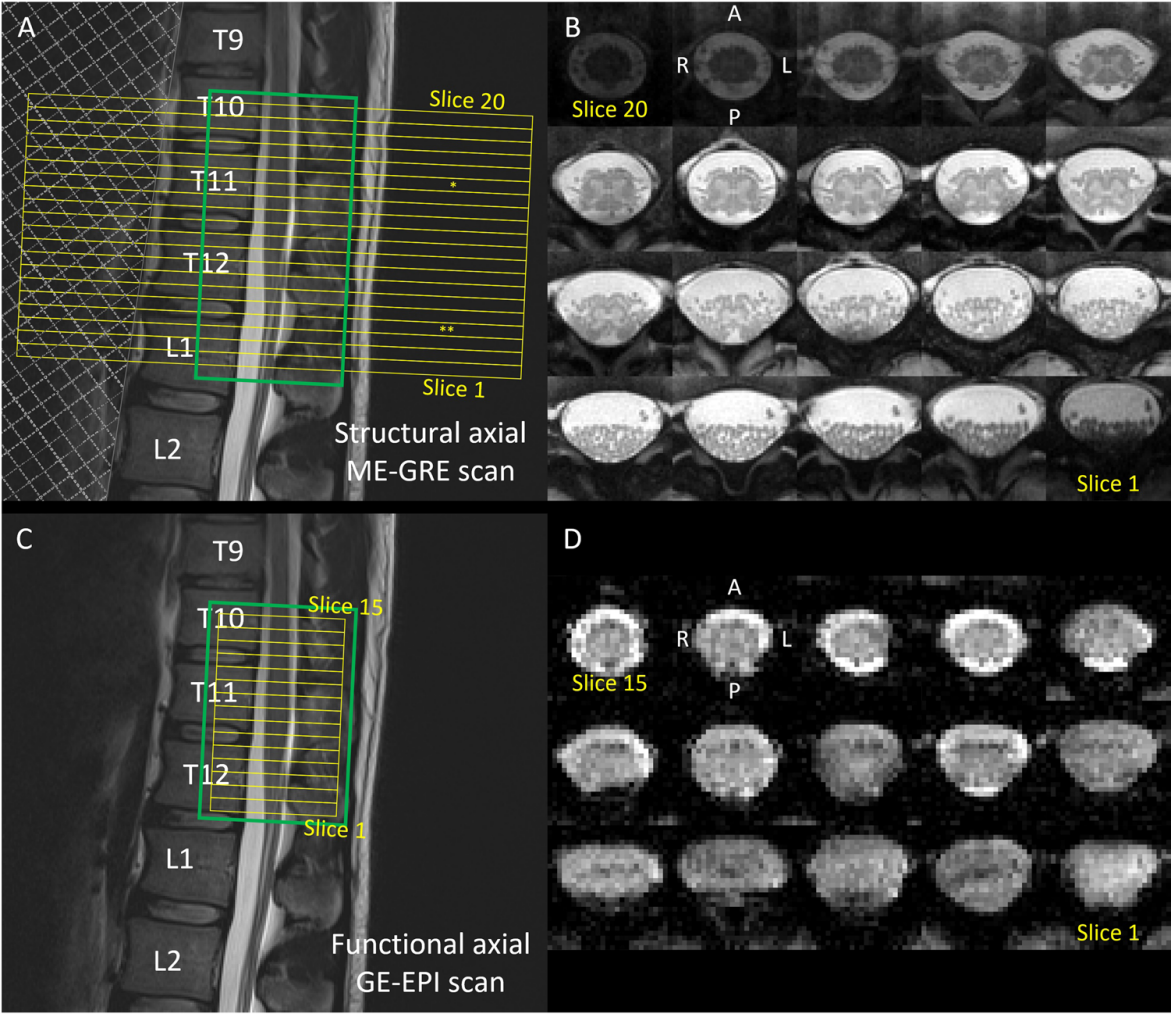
Slice positioning and example slices. Slice prescription was based on a sagittal T2-weighted turbo spin echo scan of the lower spine, for the (A) structural 3D spoiled multiecho gradient-echo (ME-GRE, Siemens FLASH) sequence and the (C) functional reduced field-of-view single-shot gradient-echo echo planar imaging (GE-EPI) sequences. The corresponding axial slices are shown for the (B) ME-GRE and (D) GE-EPI sequences (here, the iFOV35 version, seeTable 1), displayed in rostral (top left) to caudal (bottom right) direction. The slice stacks (20 slices for ME-GRE, 15 slices for GE-EPI) and shimming volumes are indicated by yellow and green boxes, respectively. The field of view (FOV) for the GE-EPI scan was set such that its sixth most rostral slice (slice #10) aligned with the maximum width of the spinal cord in the lumbosacral enlargement as observed in the sagittal T2-weighted image. The FOV for the ME-GRE scan was positioned to align the top edge with that of the GE-EPI scan (i.e., slice #20 in ME-GRE corresponds to slice #15 in GE-EPI), which ensured coverage of the lumbosacral enlargement and the conus medullaris. * indicates the slice with the largest spinal cord cross-sectional area (the “LSE landmark”) and  indicates the most caudal slice of the spinal cord (tip of the spinal cord). For the ME-GRE sequence (A), a saturation band, indicated by a shaded area, was placed anterior to the spinal column to suppress potential artifacts originating from the abdomen. Note that the OVS20 version of the GE-EPI sequence (Table 1) also included two saturation bands, placed anterior and posterior to the FOV.
